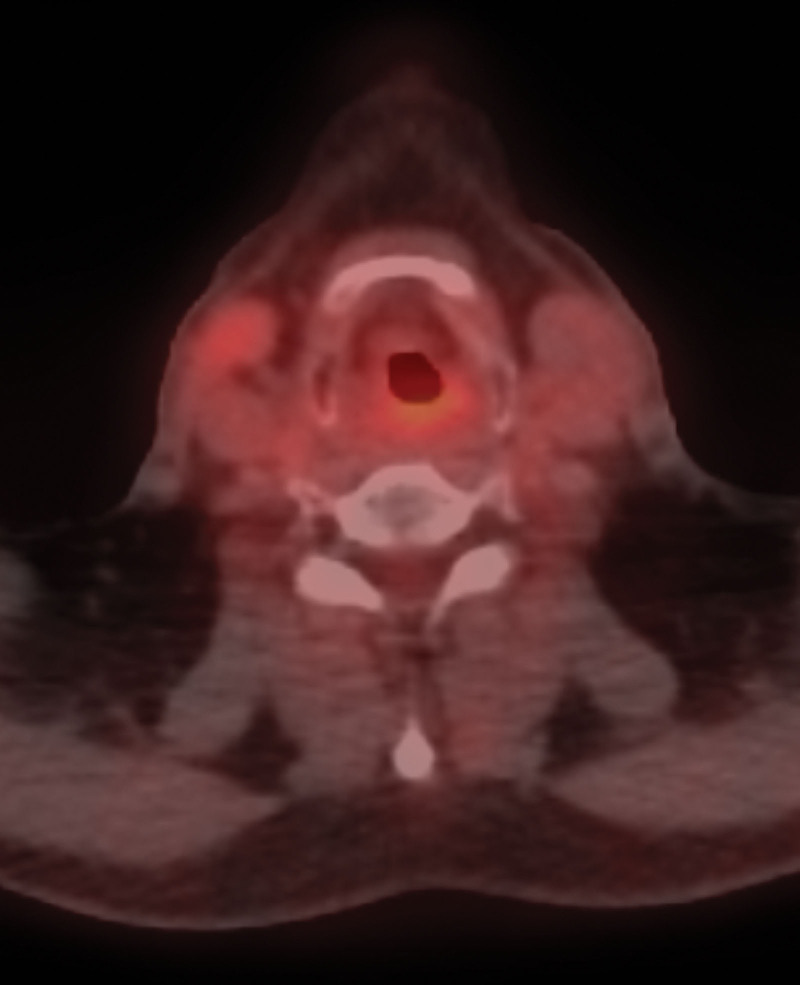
PET CT scan revealed no evidence of FDG-avid malignant lesions. CT = computed tomography, FDG = fluorodeoxyglucose, PET = positron emission tomography.
